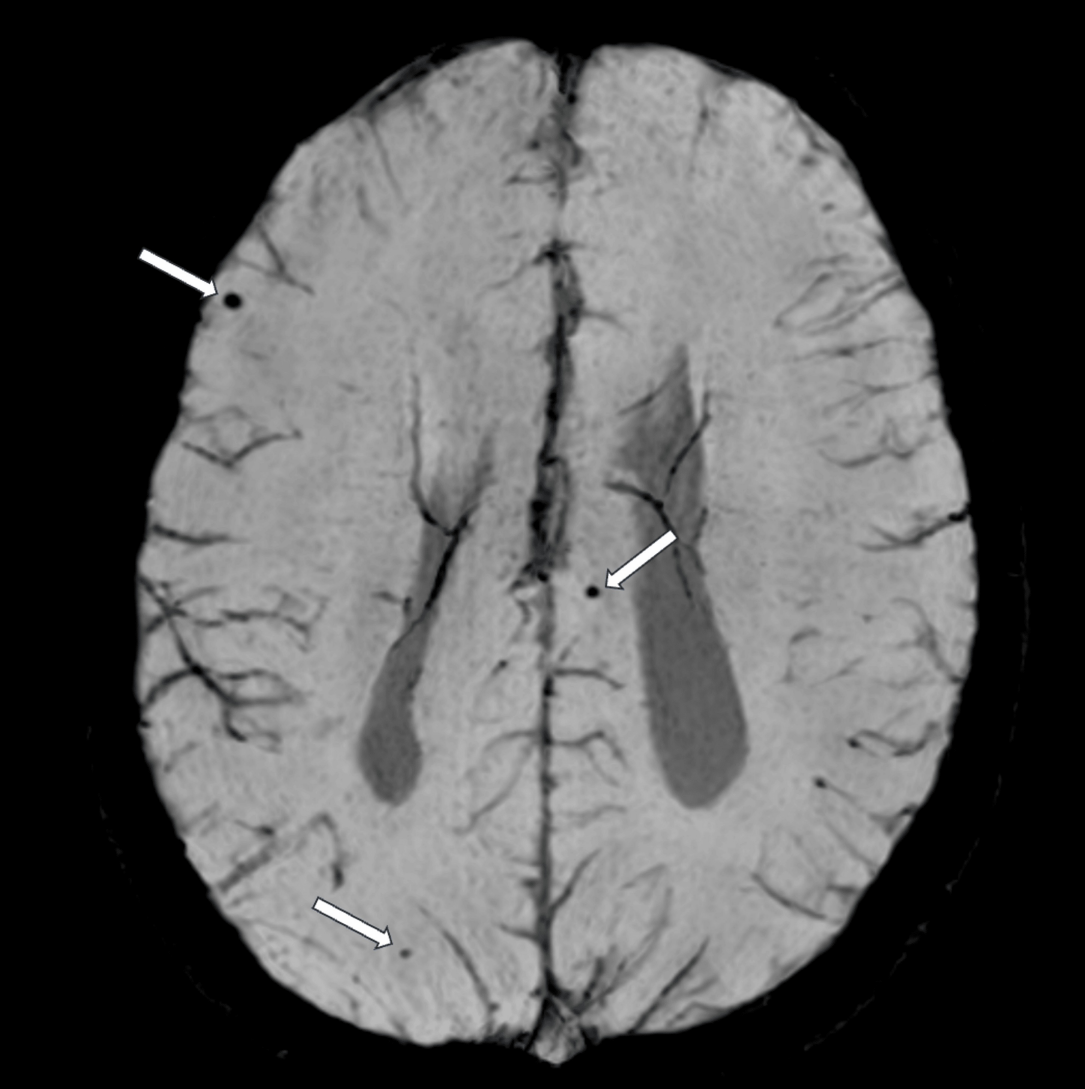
Axial-SWI MRI of the brain without contrast demonstrates punctate hemorrhages (white arrows) SWI - susceptibility-weighted imaging
